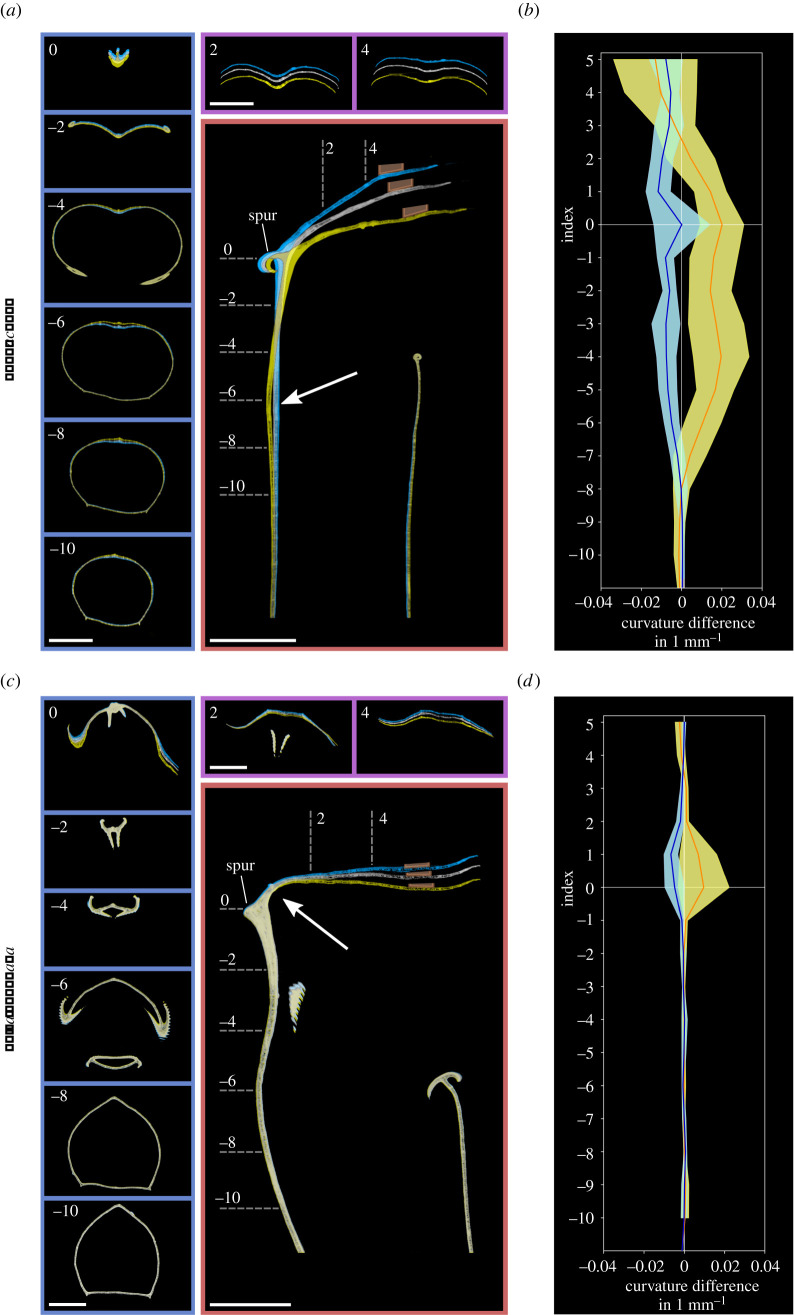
Pitcher deformation patterns for *N. gracilis* (*a*,*b*) and *N. rafflesiana* (*c*,*d*) visualized from overlays of µ-CT scans with three different lid positions (neutral, grey; downward, yellow; upward, blue). Whilst *N. gracilis* deforms mostly along the dorsal spine of the upper pitcher body, *N. rafflesiana* deforms in the basal part of the lid. (*a*,*c*) Overlaid longitudinal (red frame) and cross-sections (blue and purple frames) of the upper pitcher body and lid (scale bars = 10 mm). Arrows point to the areas of strongest deformation. The position of the manipulating skewer is indicated in the longitudinal sections. Indices mark the locations of the individual cross-sections. (*b*,*d*) Differences in the curvature of the dorsal pitcher spine between neutral and downward (orange) and upward (blue) lid positions, determined from the longitudinal sections of six *N. gracilis* (*b*) and five *N. rafflesiana* (*d*) pitchers. Lines denote means and shaded areas indicate 95% confidence intervals.
